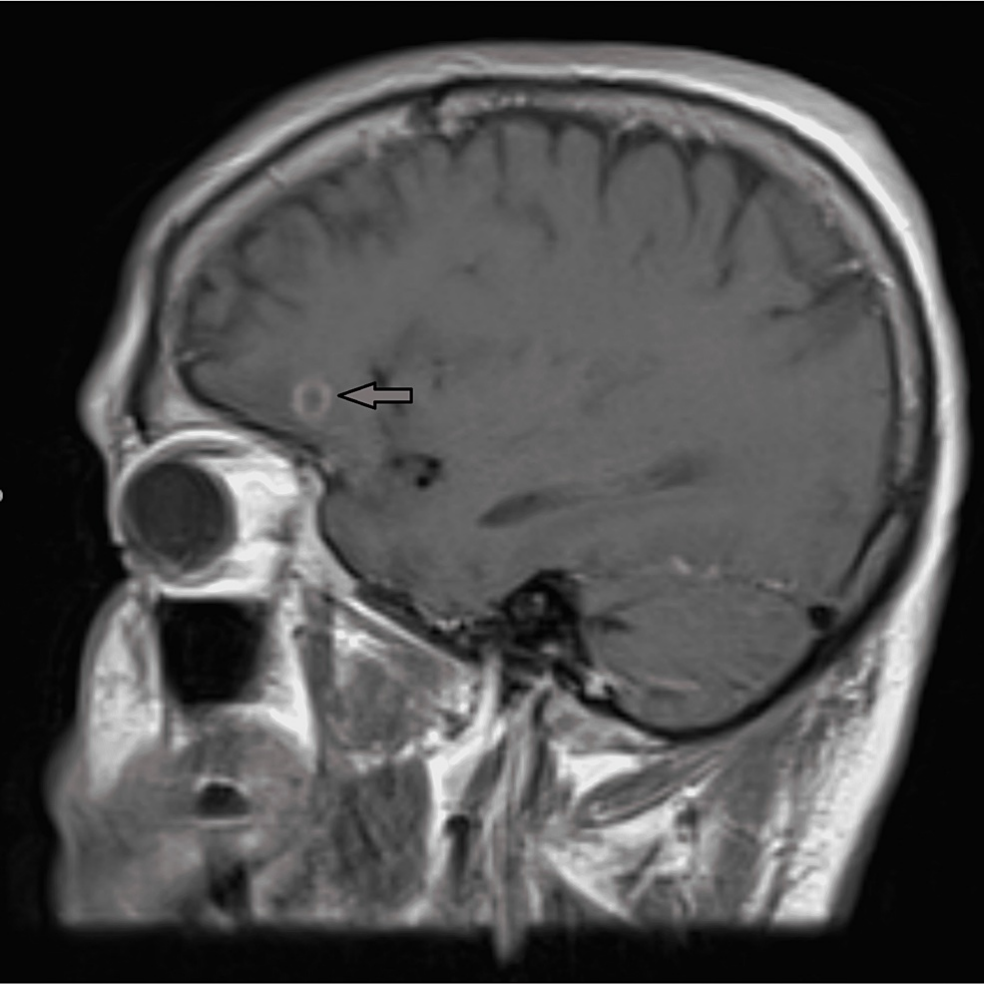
Sagittal T1-weighted magnetic resonance imaging of the initial 0.7 cm ring-enhancing lesion in the left inferior frontal cortex.
